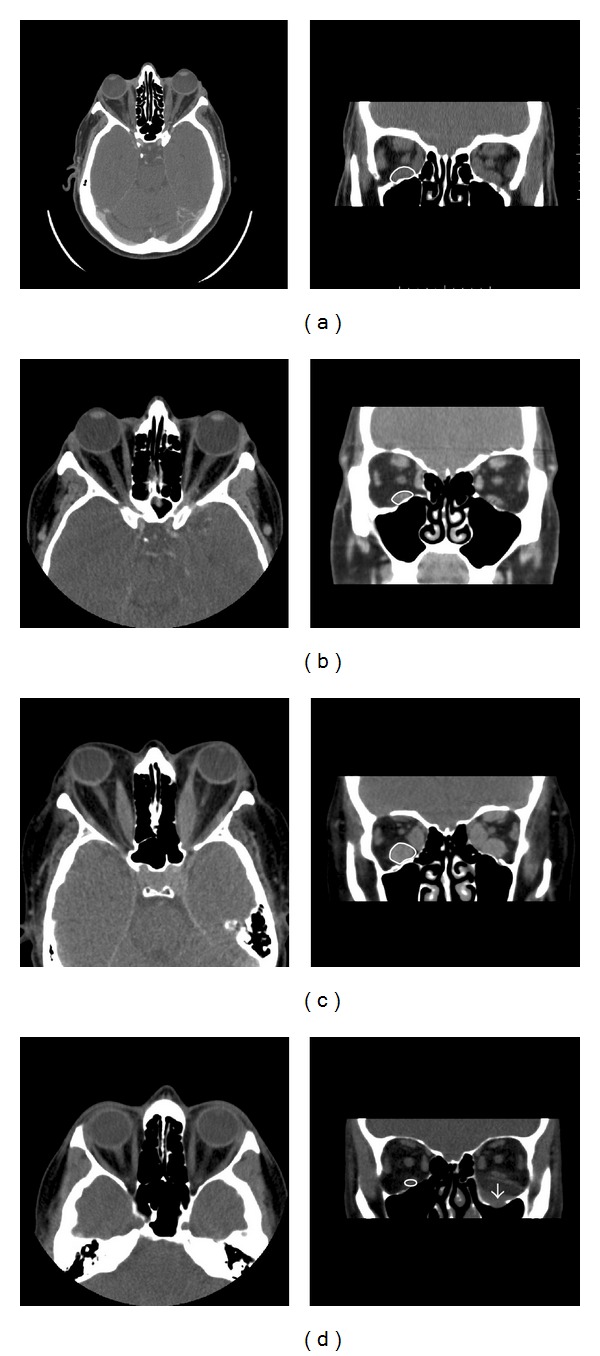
Axial and coronal CT images of 3 representative patients with Graves' disease complicated with GO ((a)–(c)) and one control patient (d). The regions of interest are drawn on each coronal slice. Patient (a) has GO with predominant muscle enlargement. Patient (b) has GO with predominant enlargement of orbital fat in the right orbit whereas the left orbit is less affected. Patient (c) has enlargement of both orbital muscles and fat in both orbits and the visual acuity was reduced to 6/18 in the left orbit as a result of DON. The visual acuity was reduced to 6/6 in the right orbit. The patient shown in (d) had a floor fracture in the left orbit and the right orbit served as one of the 13 normal controls.
